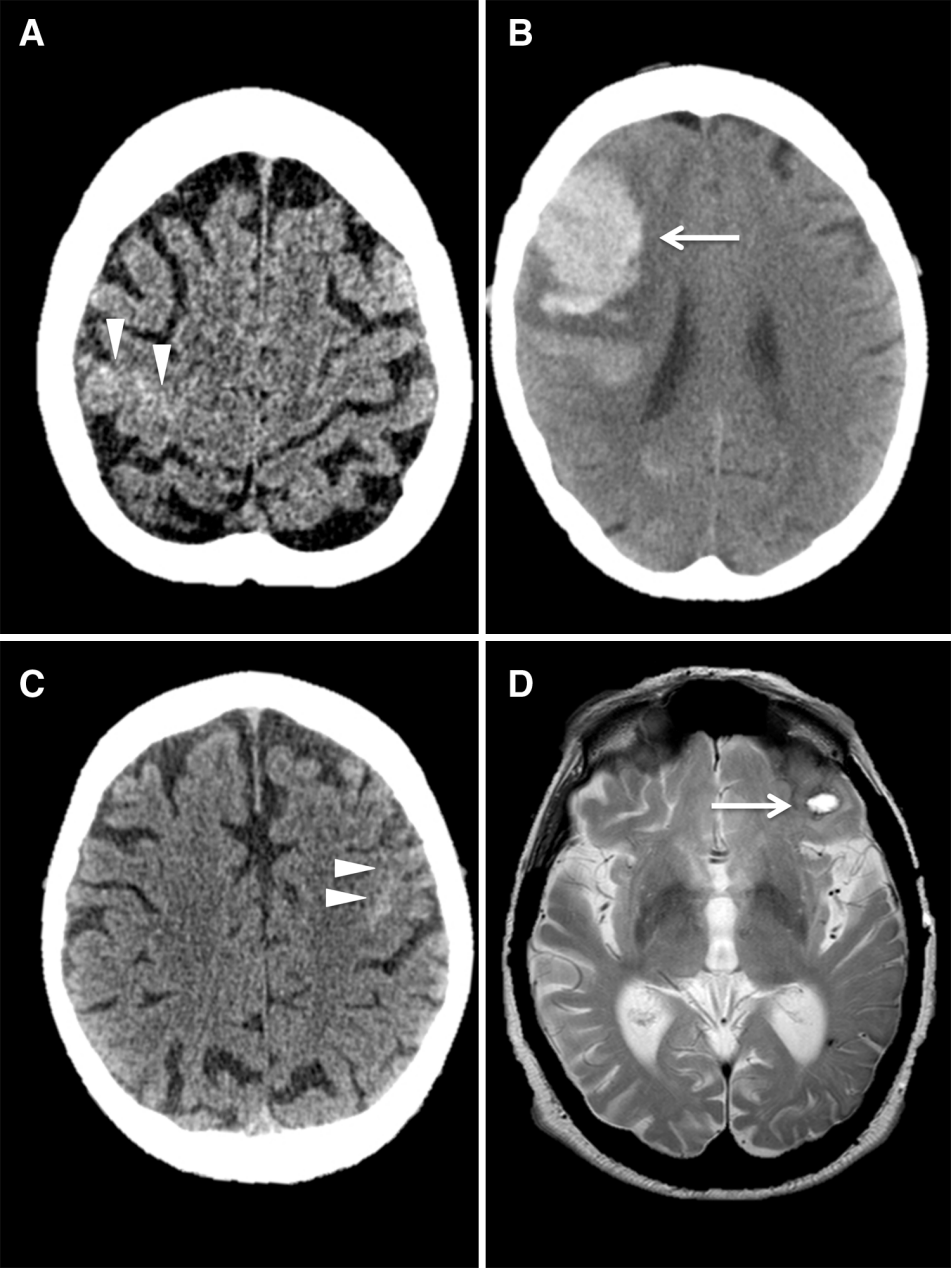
a Axial CT scan shows acute cSAH in the right central sulcus (*white arrowheads*); b follow-up axial CT shows a subsequent right frontal ICH in the same patient as *panel* (a) (*white arrow*); c axial CT scan shows acute cSAH in a left frontal lobe sulcus (*white arrowheads*); d a follow-up axial T2-weighted MRI shows an acute left frontal ICH in the same patient as *panel* (c) (*white arrow*)
